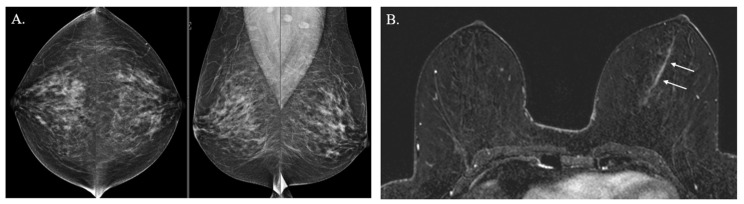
A 53-year-old female with TCv8 lifetime risk of 22.8% secondary to breast density and family history undergoing screening mammography and supplemental screening in a staggered regimen. (A) Bilateral screening mammography (CC and MLO views) shows category C density (heterogeneously dense) without mammographic abnormality. (B) Breast MRI, axial post-contrast, subtraction image shows 6.4 cm of linear non-mass enhancement in the central left breast (arrows). Biopsy reveals intermediate-grade DCIS with comedo necrosis.
